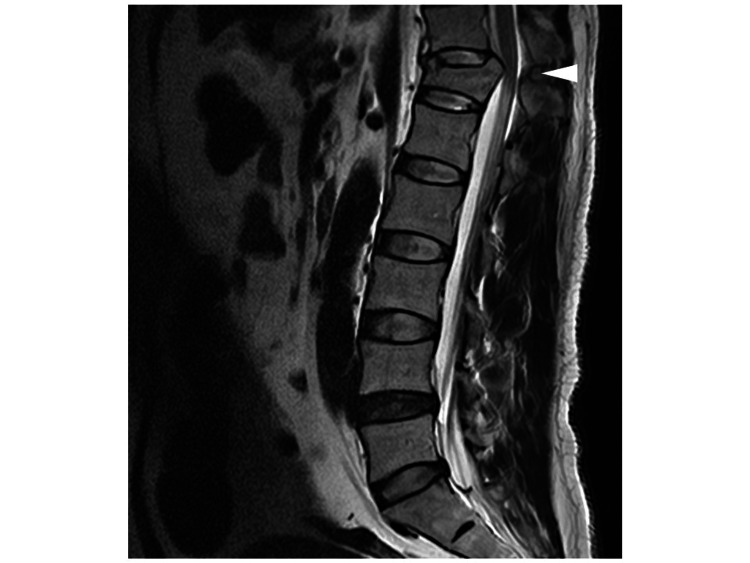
A spinal cord magnetic resonance T2-weighted image showing a burst fracture of the 12th thoracic vertebra The arrowhead indicates a burst fracture.
